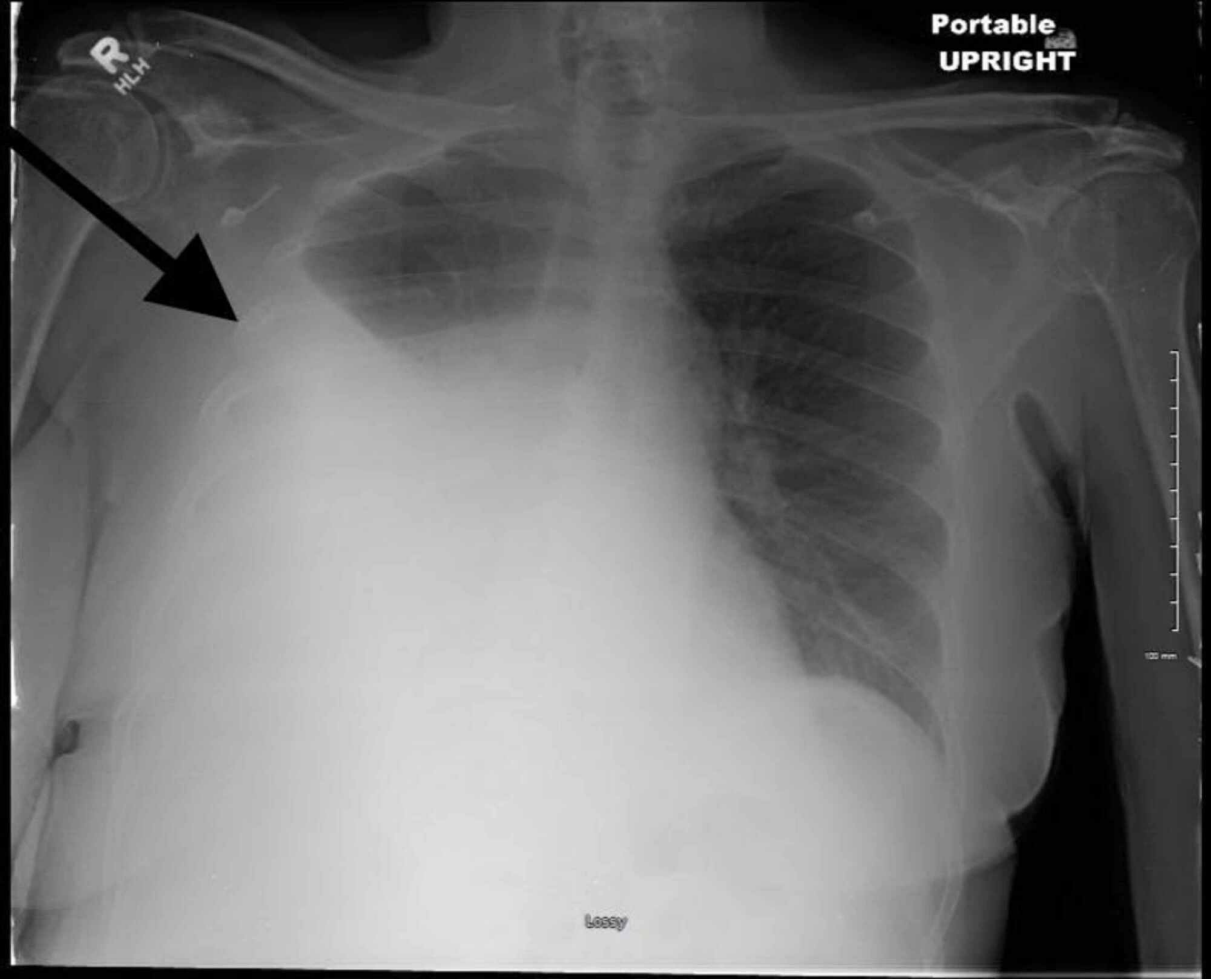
Chest X-ray showing large right-sided pleural effusion.
